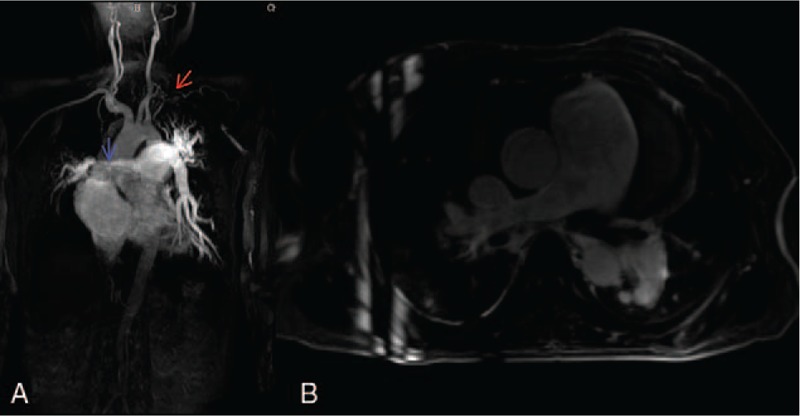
A 32-year-old woman with the stable Takayasu arteritis. Magnetic resonance angiography (MRA) (A) showed the stenotic left subclavian artery (red arrow) and right pulmonary artery (blue arrow). DCE-MRI with three-dimensional inversion recovery Turbo fast low-angle shot (3D IR Turbo FLASH) (B) did not show delay enhancement in the arterial walls.
